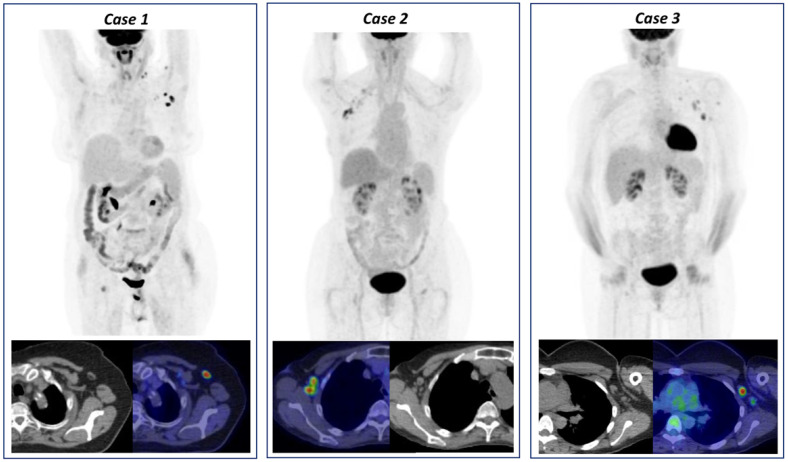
Three cases of vaccine-associated hypermetabolic lymphadenopathy (VAHL) detected on [^18^F]FDG PET/CT. Case 1: [^18^F]FDG PET/CT performed in a 67-year-old woman for left breast cancer follow-up and increased tumor markers. The images showed [^18^F]FDG-uptake in left supra- and retro-clavicular lymph nodes (maximum standardized uptake value, SUVmax 3.3) as well as homolateral axillary lymphadenopathy (SUVmax 7.0), classified as grade 3. Patient medical history reported the booster vaccine dose injection ipsilateral to PET findings 7 days before examination. Case 2: [^18^F]FDG PET/CT performed in a 42-year -old woman for melanoma of right dorsal region follow-up. The images showed [^18^F]FDG-uptake in right subclavicular and interpectoral lymph nodes (SUVmax 3.9), classified as grade 2. Patient medical history reported the booster vaccine dose injection ipsilateral to PET findings 16 days before examination. Case 3: [^18^F]FDG PET/CT performed in a 80-year-old man for follow-up of superior diaphragmatic non-Hodgkin lymphoma. The images showed [^18^F]FDG-uptake in left cervical, subclavicular and axillary lymph nodes (SUVmax 14.5), classified as grade 3. Patient medical history reported the booster vaccine dose injection ipsilateral to PET findings 2 days before examination. All these findings were VAHL, considering clinical history and the lymph nodes features on co-registered CT images.
